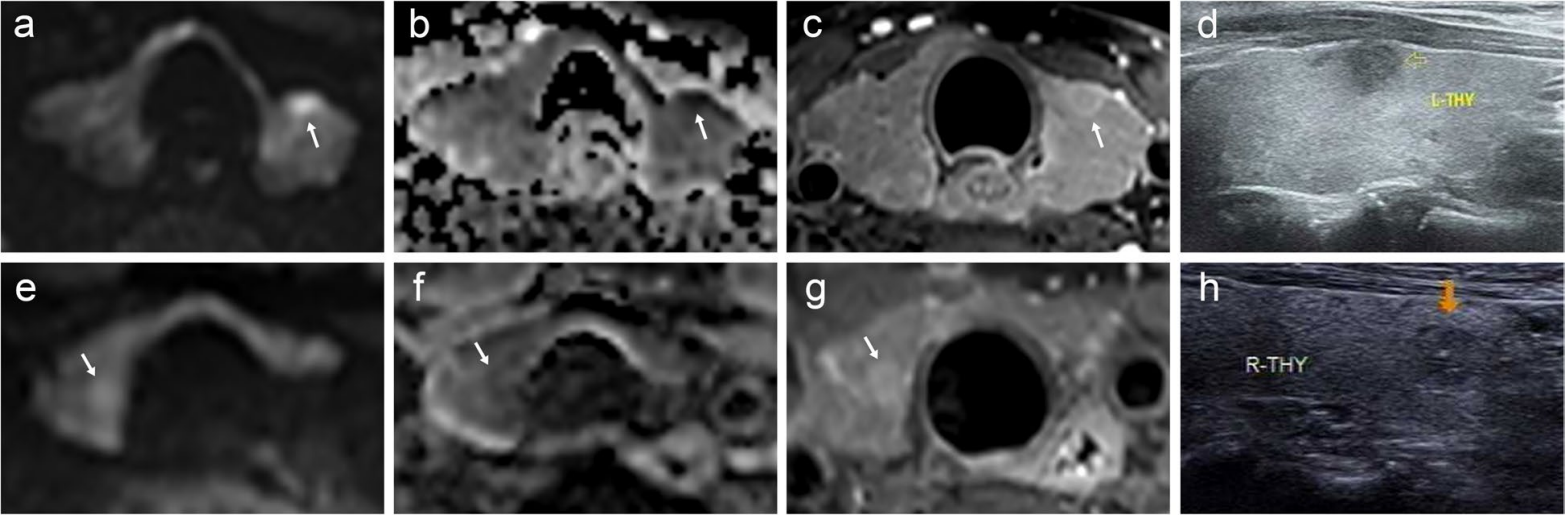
A 51-year-old female with papillary thyroid carcinoma in the left lobe (a, b, c and d). Axial diffusion-weighted imaging (DWI) (a) and apparent diffusion coefficient (ADC) map (b) show restricted diffusion of the lesion (white arrow). The delayed phase contrast-enhanced T1-weighted imaging (c) shows reversed halo sign (white arrow). The ultrasound image (d) displays a lesion initially classified as ACR-TIRADS grade TI-RADS 4, which has been upgraded to the improved ACR-TIRADS grade of TI-RADS 5, indicating the recommendation for fine needle aspiration biopsy. A 67-year-old female with adenomatous goiter in the right lobe (e, f, g and h). Axial DWI (e) and ADC (f) show absence of restricted diffusion (white arrow). The delayed phase contrast-enhanced T1-weighted imaging (g) shows a relatively homogeneous enhancement pattern (white arrow). The ultrasound image (H) of the lesion, initially classified as ACR-TIRADS grade TI-RADS 4 but subsequently revised to an improved ACR-TIRADS grade TI-RADS 3, obviating biopsy
